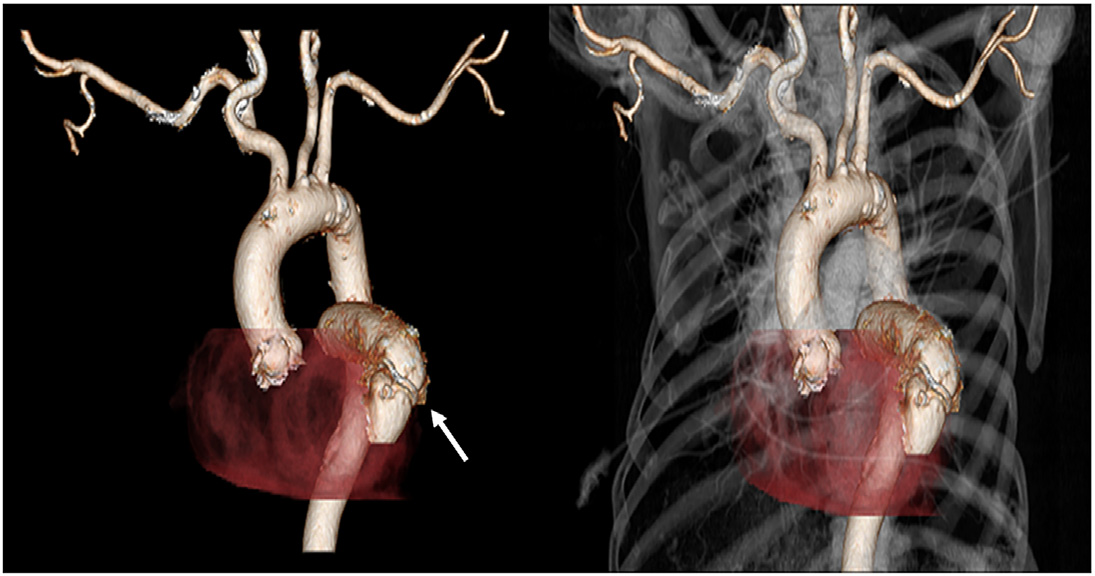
Postoperative computed tomography angiogram of the chest demonstrates a widely patent left atrial to left ventricular bypass. Note the proximity of the prosthetic valve to the ventricular anastomosis (arrow).
